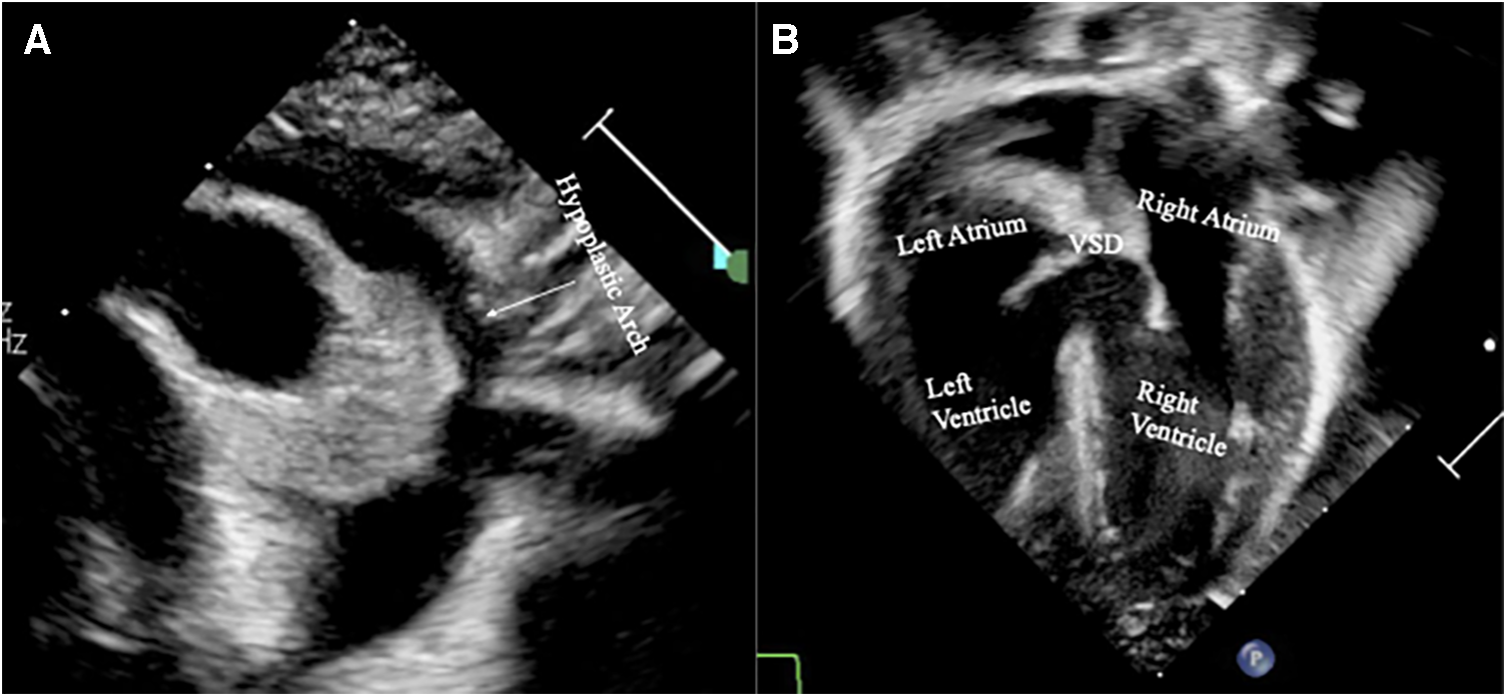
Echocardiographic images obtained shortly after birth demonstrating (A) a severe hypoplasia of the ascending aorta and its arch, and (B) a substantial muscular ventricular septal defect (VSD).
